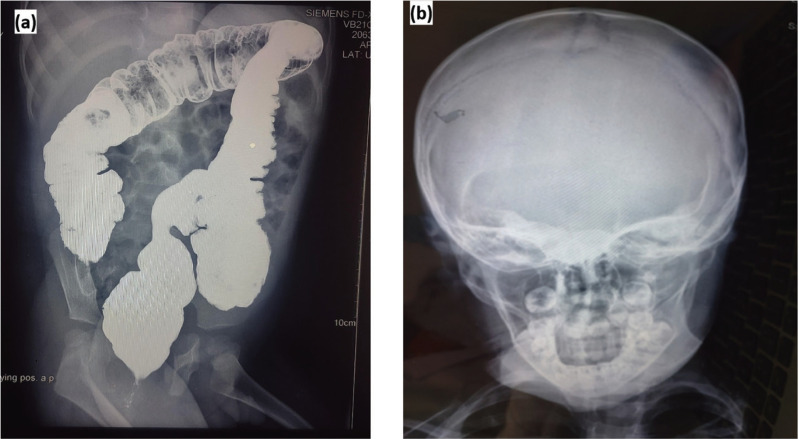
(a) Radiograph during barium enema procedure suggestive of Hirschsprung disease, (b) Skull AP view showing dolichocephaly.
